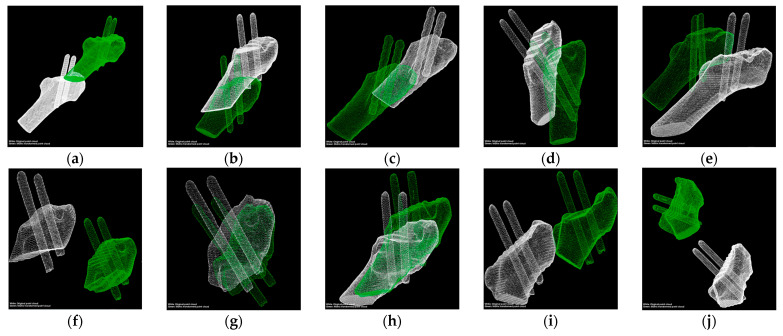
3D point clouds preprocessing. (a–j) are the point clouds of the 10 cases without performing alignment, respectively. The white point cloud is generated based on postoperative CT images, and the green point cloud is generated based on CT images scanned after the one-year recovery period.
